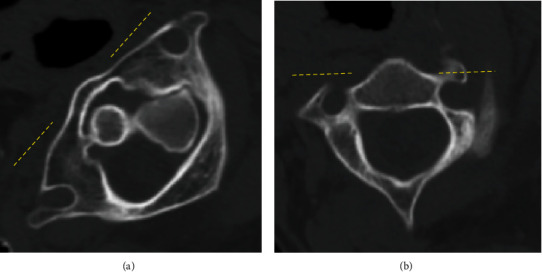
CT showing recurrence of AARF. (a) Axial view of C1 and (b) axial view of C2. The rotatory angle increased to 45 degrees.
